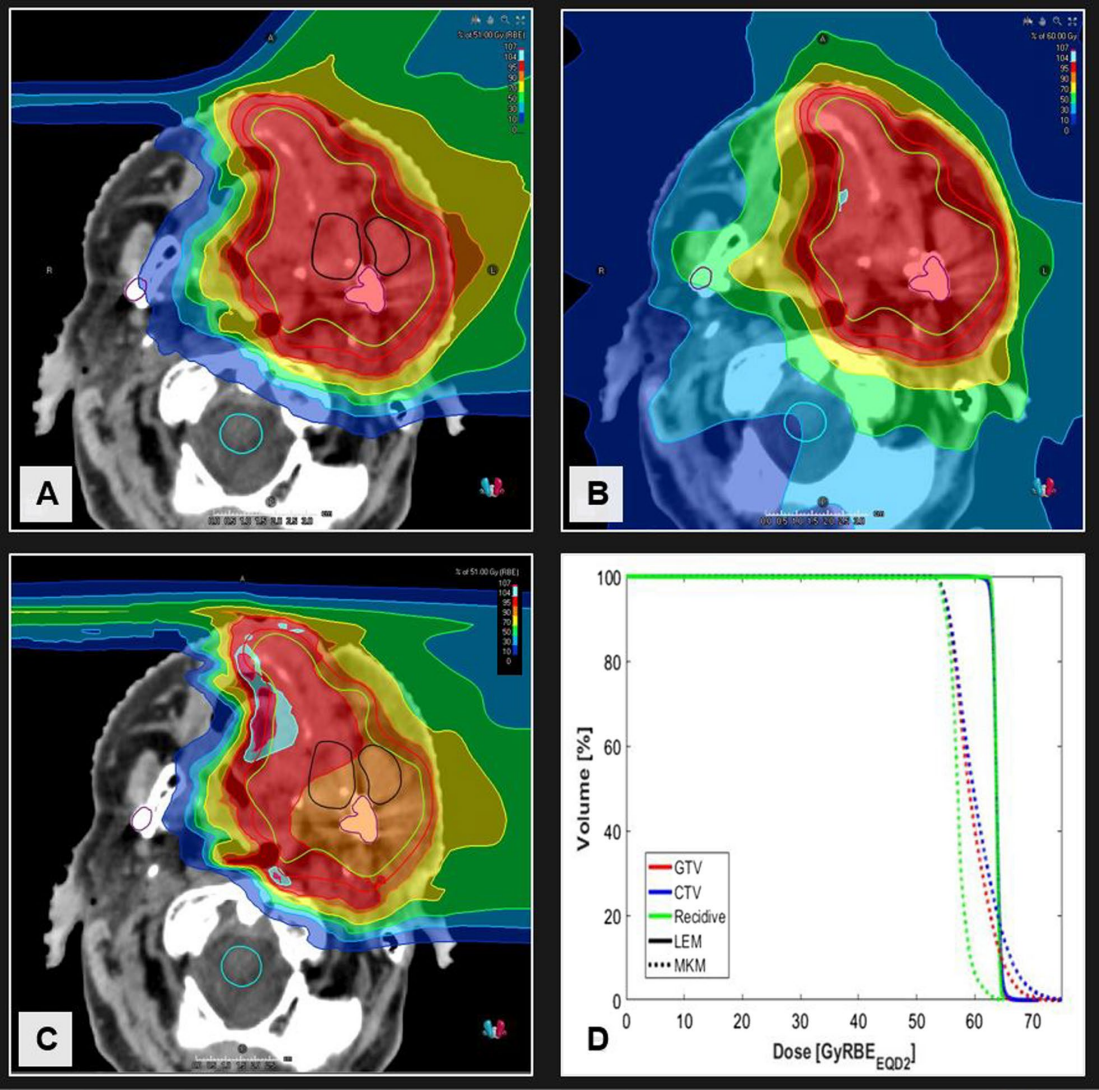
69-year-old female patient with recurrent oral cavity cancer treated with 51 Gy (RBE) CIRT (A) around 28 years after prior radiotherapy with 75 Gy. CIRT yielded significant clinical benefits w.r.t. the brainstem (− 47.5% Dmax), ipsilateral optic nerve (− 16.8% Dmax), ipsilateral eye (− 21.3% Dmean) and contralateral inner ear (− 23.2% Dmean) compared to VMAT (B). The patient developed type A local failure, delineated on the planning CT (A), caused biological and/or dosimetric failure. Dose recalculation with the modified microdosimetric model w.r.t. the type A failure revealed significantly reduced rGTV Dmean and rGTV D95% dose EQD2 compared to the local effect model I (C–D)
